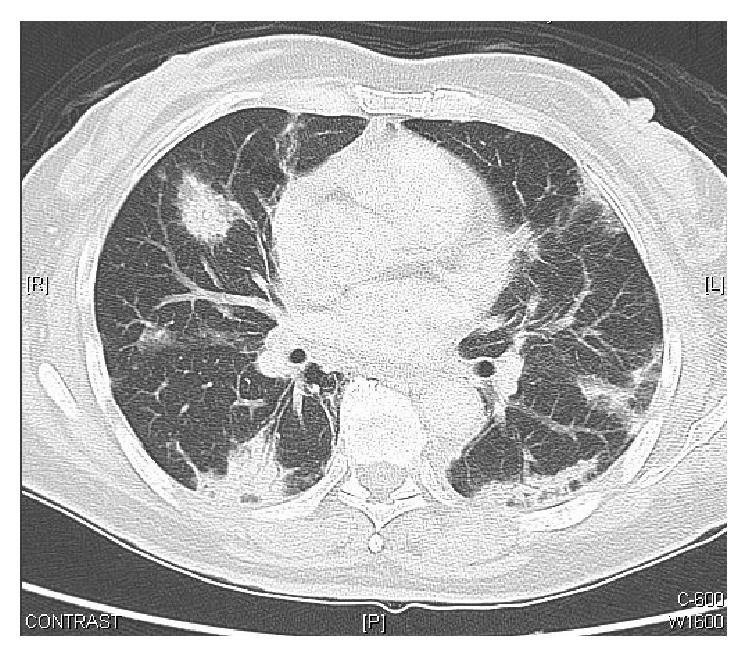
High-resolution computed tomography scans show peribronchial and subpleural bilateral ground-glass opacities with air bronchograms, some with pleural-based triangular shape.
